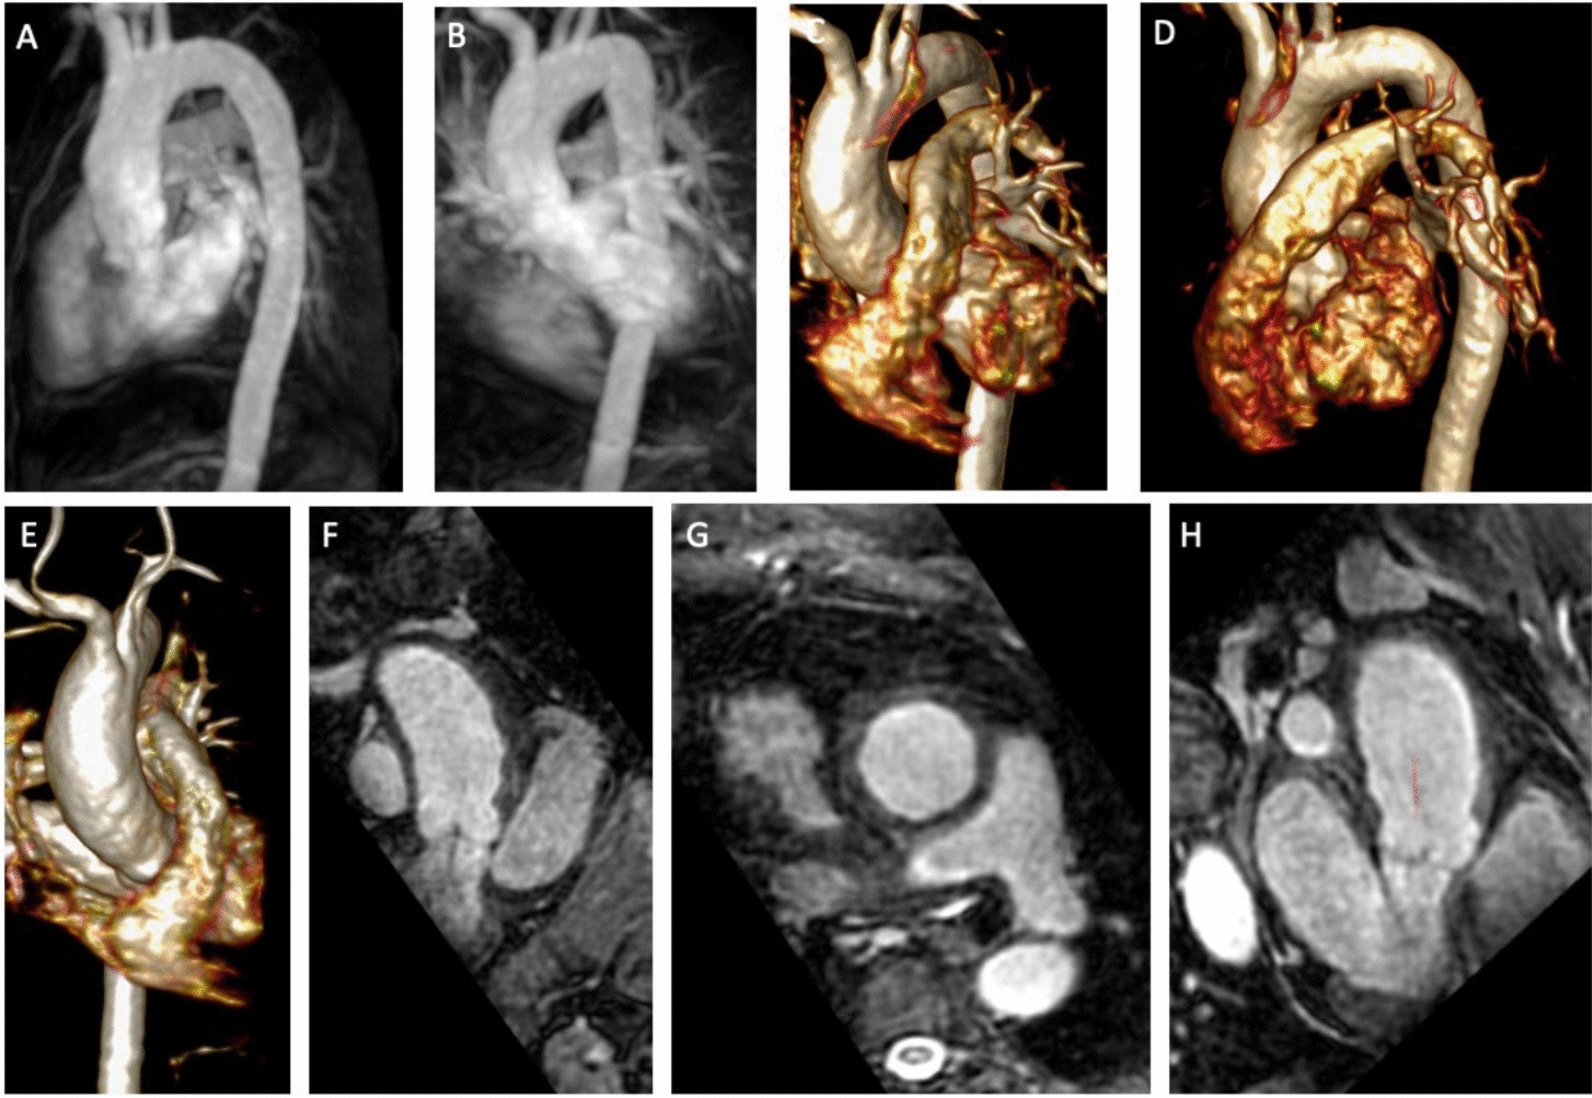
Preoperative multidirectional images of CTA and MRI. A, B Preoperative 3D contrast enhanced MIP; C–E 3D contrast enhanced mRA volume rendering; F–H 3D non contrast MRA
